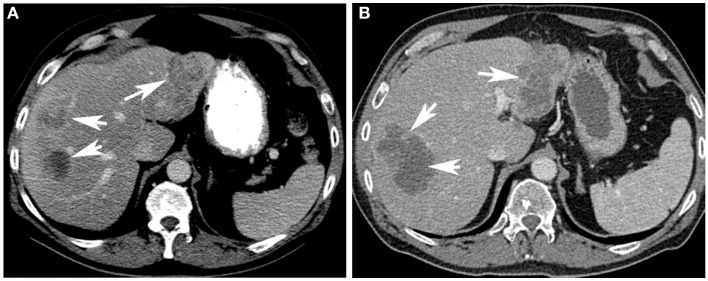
Assessment of response to Y-90 therapy on CT. A contrast enhanced CT scan (A) of the liver demonstrates hypo-attenuating lesions (arrows) in the liver. A repeat contrast enhanced CT scan (B) obtained 6 weeks after Y-90 therapy shows very low attenuation of the lesions (arrows). Even though the lesions size has not significantly changed, the development of very low attenuation on post-treatment scan suggests response to Y-90 therapy.
